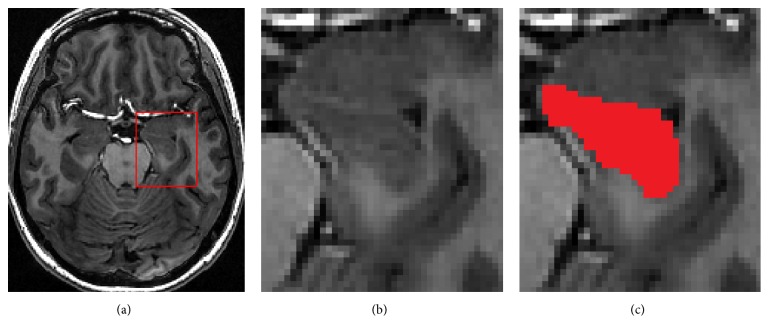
Position and intensity of hippocampus in MR image. (a) Original image of hippocampus with a rectangle; (b) zoomed view of the rectangle region; (c) zoomed view of the region where the hippocampus is indicated with red color.
